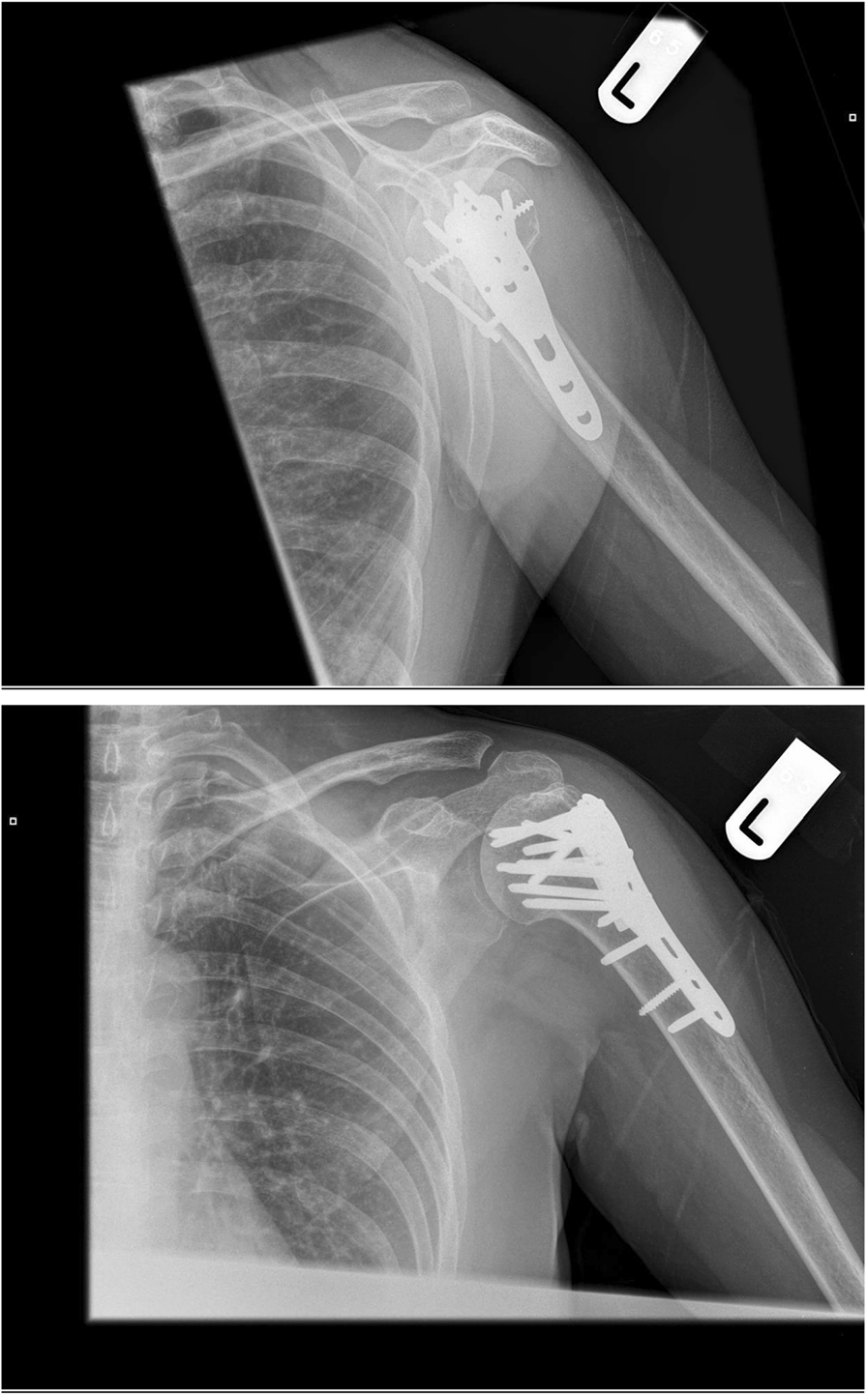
Radiographs at 1 year post-operatively
